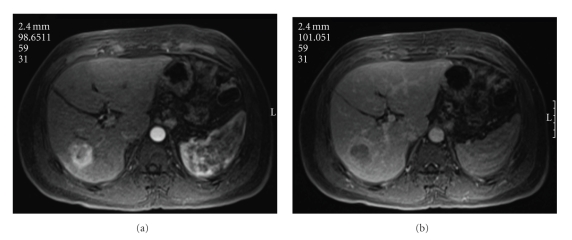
Magnetic Resonance Imaging with arterial and venous phases demonstrating a mass in segment 6 consistent with hepatocellular carcinoma.
